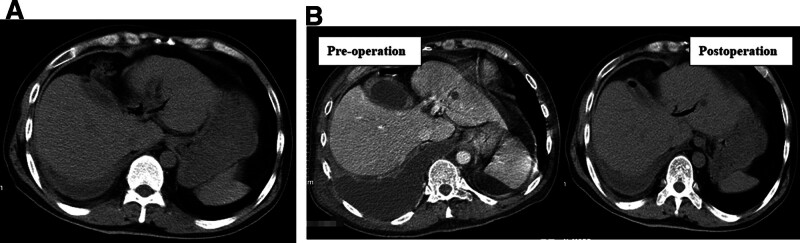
(A) Computed tomography (CT) examination 20 d after surgery indicated that most pleural effusions was absorbed, gallbladder volume was reduced, and perigallbladder inflammation was gradually absorbed. (B) Compared with preoperative and postoperative CT scans, perigallbladder inflammation and pleural effusion were significantly reduced. Due to the placement of bile duct stents in the bile duct, there was a little air accumulation in the left intrahepatic bile duct and gallbladder after surgery.
